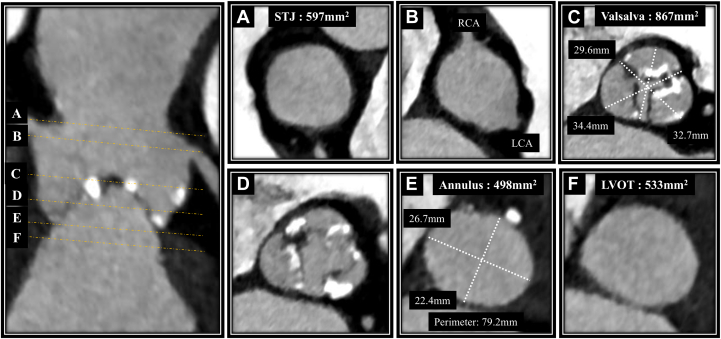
Preoperative Computed Tomography Findings of the Aortic Valve (A) Sinotubular junction (STJ). (B) The ostium of the left coronary artery (LCA) and right coronary artery (RCA). (C and D) Sinus of Valsalva. (E) Annlus. (F) Left ventricular outflow tract (LVOT).
